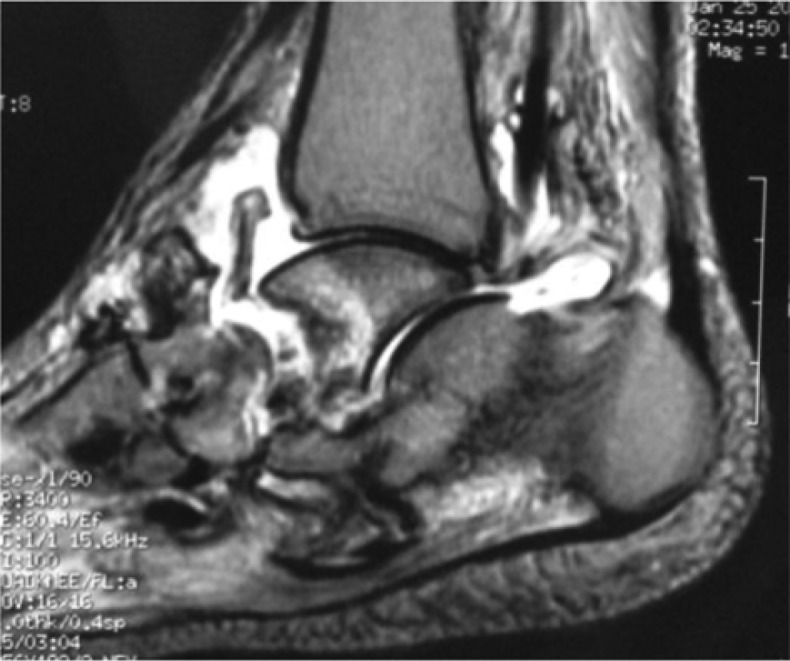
Sagittal view of a magnetic resonance imaging showing the ankle anterior capsule breached, soft tissue oedema and bone oedema in the anterior talar body.
